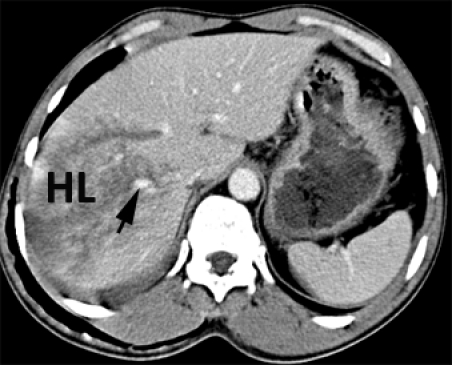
Abdominal CT. Degree Ⅳ liver laceration. Arrow – right hepatic vein.
